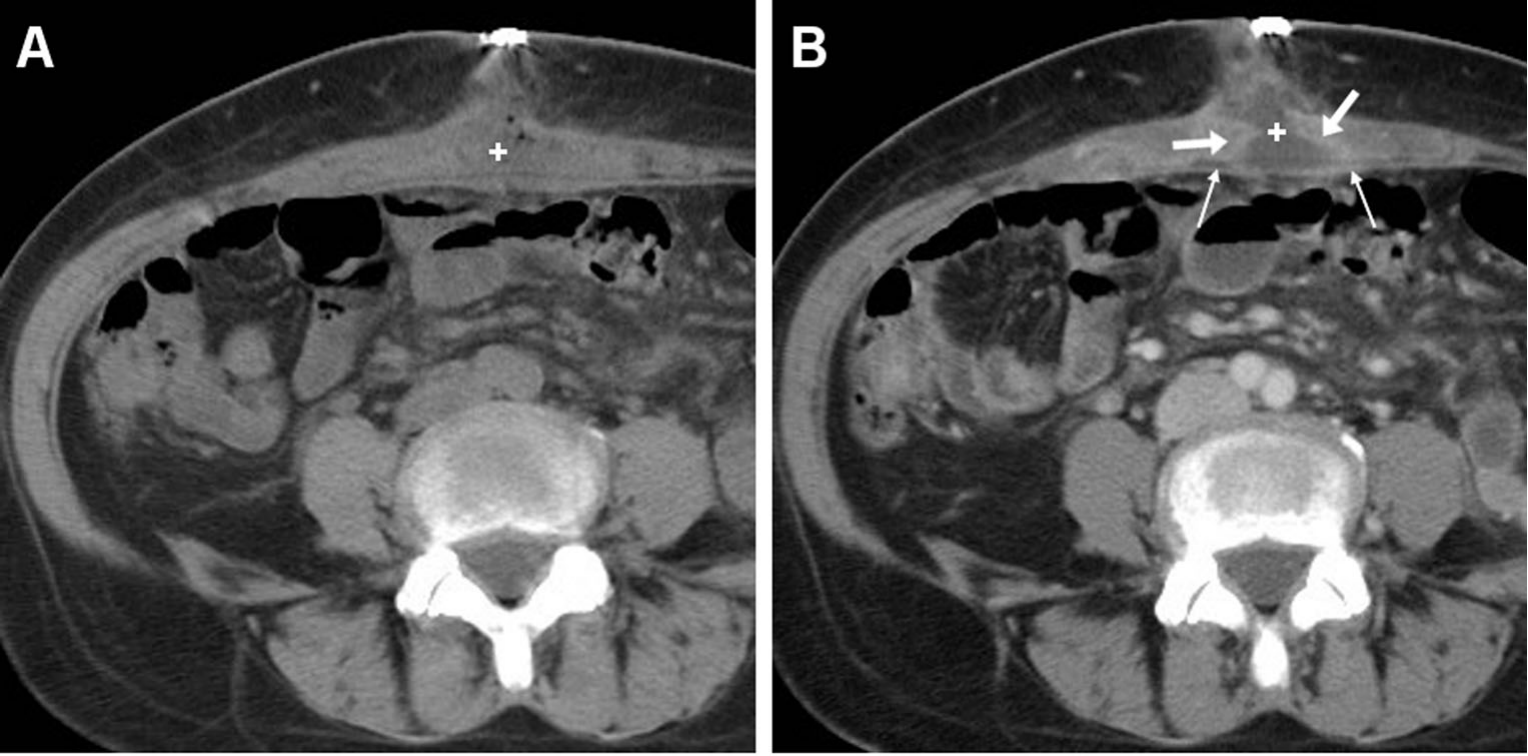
Common appearance of postoperative seroma detected six days after open ventral hernia repair (VHR) in a 51-year-old female. Unenhanced (a) and post-contrast (b) axial CT images showed a small-sized midline fluid-attenuating collection (+) containing tiny gas bubbles, interposed between the rectus muscles, abutting the PM-reinforced parietal peritoneum indicated by a linear structure isoattenuating to muscles (thin arrows). In the absence of clinical and laboratory signs of infection, thin peripheral contrast enhancement (arrows in b) was observed. The seroma eventually resolved without any additional treatment
